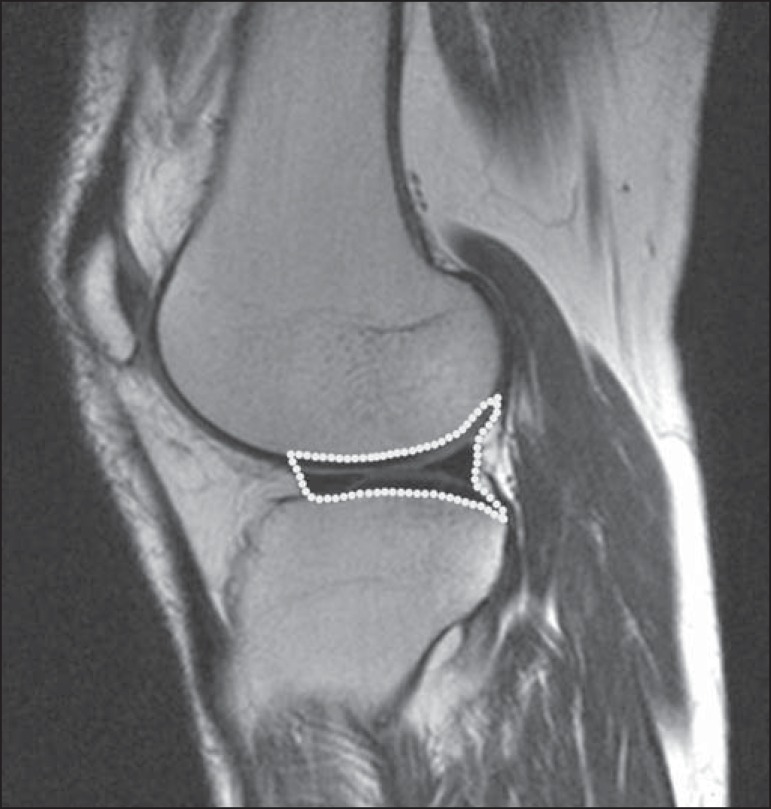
Magnetic resonance imaging scans of the *in vivo*
